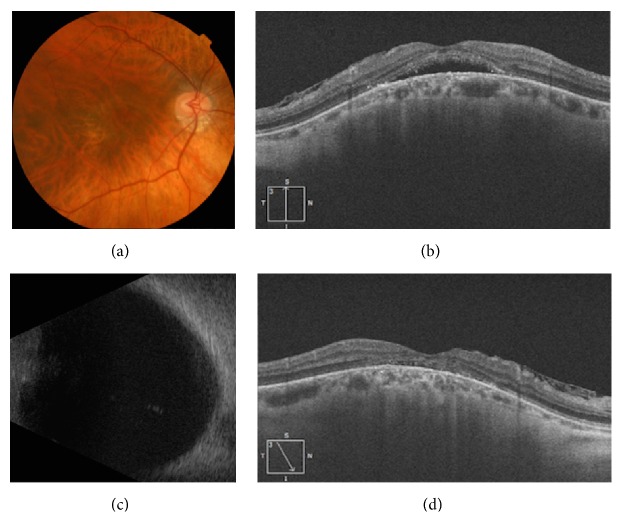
Staphyloma edge with serous retinal detachment before and after treatment with spironolactone. (a) Color photo showing a tilted-disc with a type 5 staphyloma described by Curtin [2], inferior peripapillary atrophy, marked retinal thinning with visible choroidal vessel, but no hemorrhage in the foveal area. (b) Pretreatment OCT image showing serous retinal detachment in the foveal area with associated epiretinal membrane. (c) B-scan ultrasound showing an abnormal ocular wall in the posterior fundus. (d) Posttreatment OCT image showing resolution of the serous retinal detachment in the foveal area with an abnormal ellipsoid line and associated epiretinal membrane.
